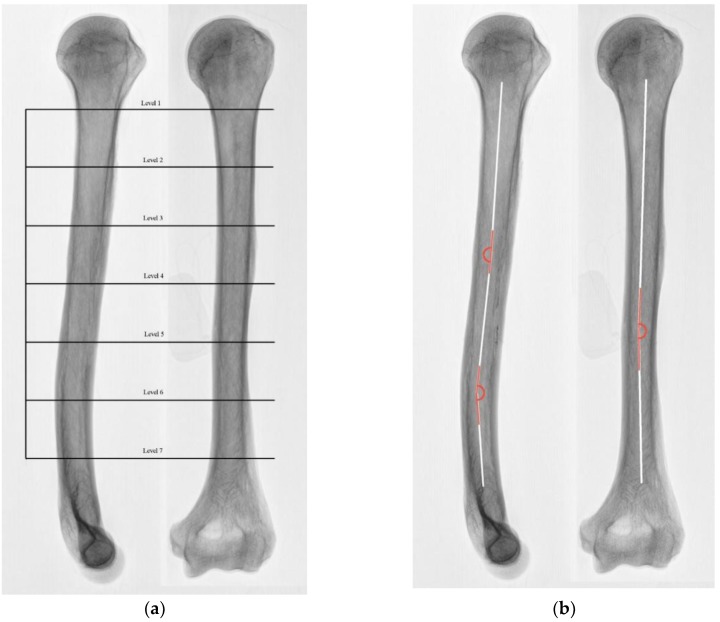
Left humerus, lateral (left) and anteroposterior (AP) radiograph (right). (a) Measurement levels from the surgical neck to the beginning of the medial supracondylar ridge are marked in black as 1–7. (b) The axis of the medullary canal is marked in white, and the corresponding angles are marked in red. While the slight valgus bending seems irrelevant, pro- and especially recurvatum angles should be considered both in ante- and retrograde nailing.
